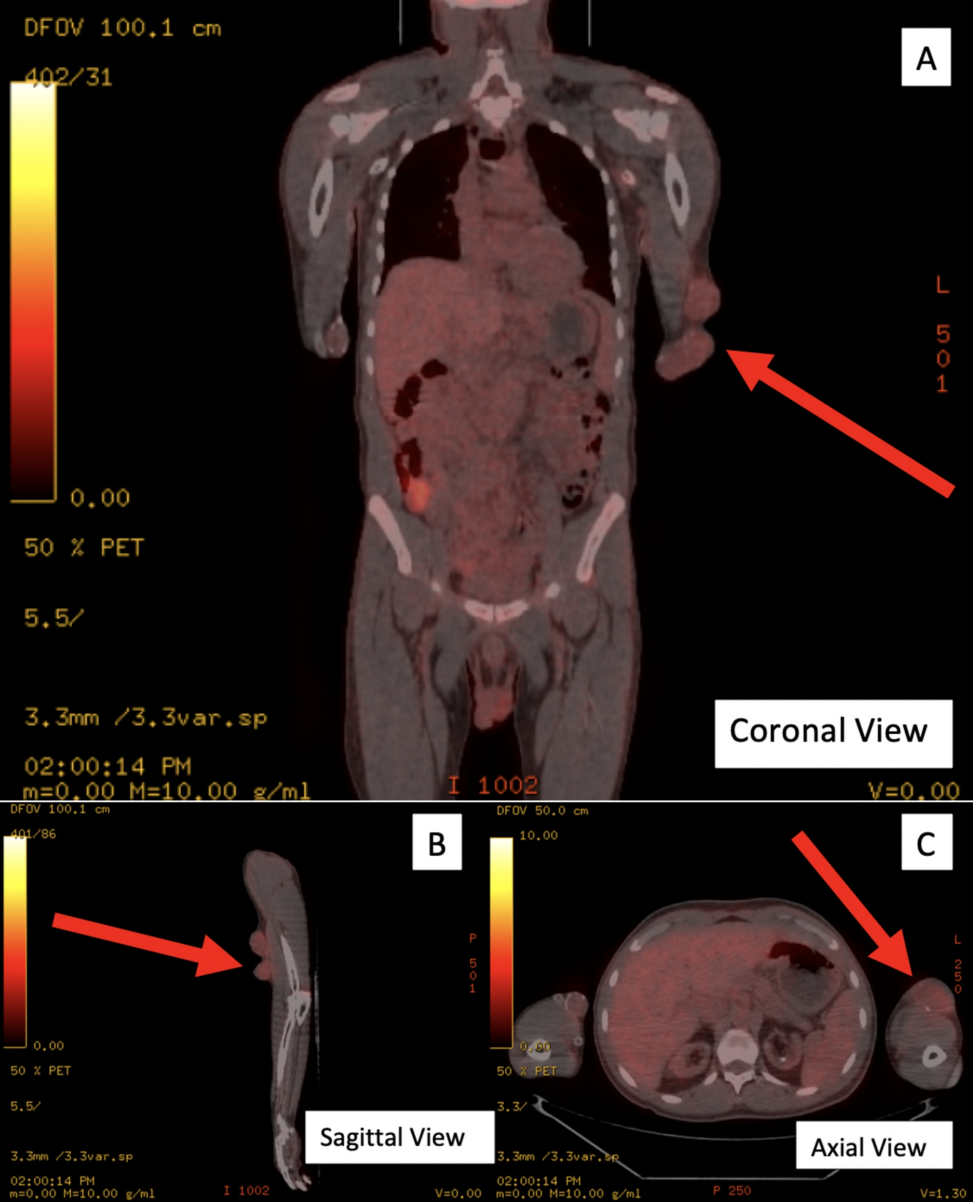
F-18-Fluorodeoxyglucose Positron Emission Tomography/Computerized Tomography (FDG PET/CT) Scan FDG PET/CT scan revealed abnormal FDG uptake suspicious for infection and inflammation at the left upper extremity fistula with highest suspicions at proximal subcutaneous. Scan includes coronal (A), sagittal (B), and axial (C) views. The red arrows denote site of increased FDG uptake.
